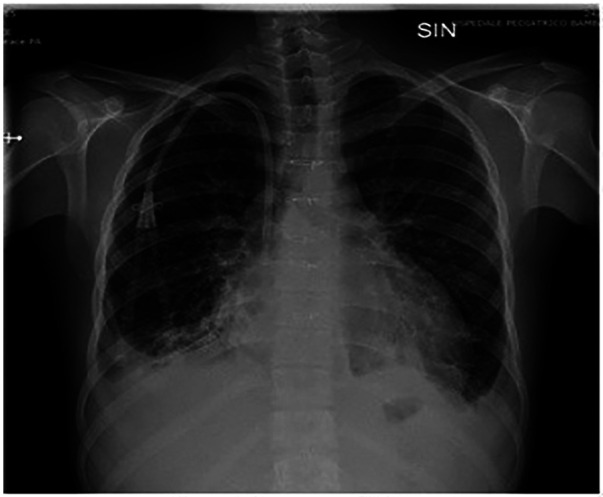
Chest radiograph shows bilateral pleural effusion.
